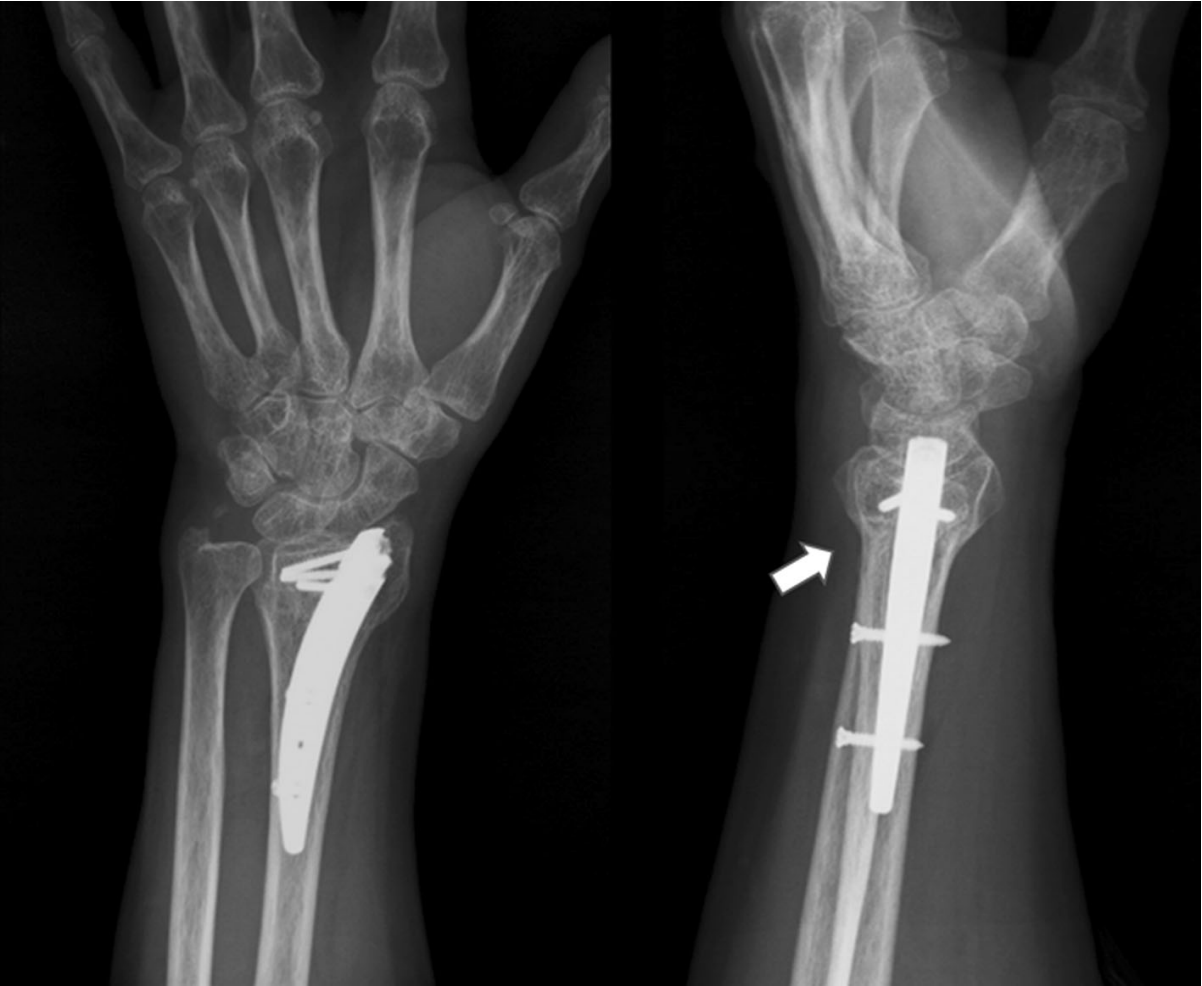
Radiographs at 6 months after surgery show osseous union and consolidation of osteotomy site (*white arrow*)
